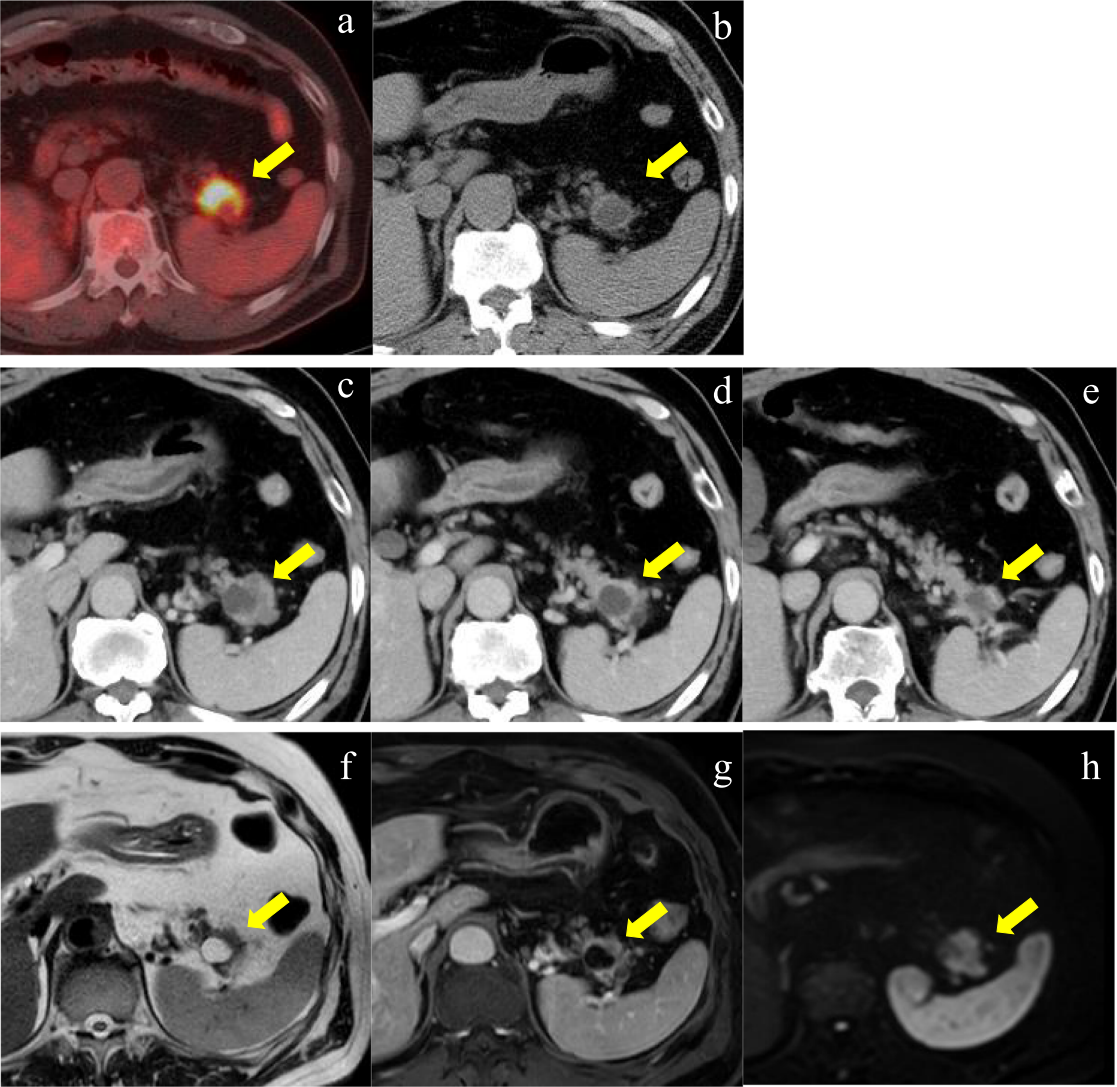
Preoperative images of the pancreatic tumor. a PET-CT before pembrolizumab therapy shows a solitary mass in the splenic hilum (arrow; SUVmax: 12.8). b Abdominal CT before pembrolizumab therapy shows a low-density mass in the splenic hilum, about 20 mm in diameter (arrow). c-e A series of contrast-enhanced CT scans after pembrolizumab therapy. The mass shows slight expansion up to 25 mm in size. This tumor is located in the tail of the pancreas, including a cystic lesion surrounded by a solid component that is irregularly enhanced in the delayed phase (arrows). f-h Magnetic resonance imaging (MRI) after pembrolizumab therapy. The tumor (arrows) is composed of a high-intensity moiety on the T2-weighted image (f), and surrounding parenchyma is enhanced by contrast medium in the delayed phase (g) and shows high intensity on the diffusion-weighted image (h)
